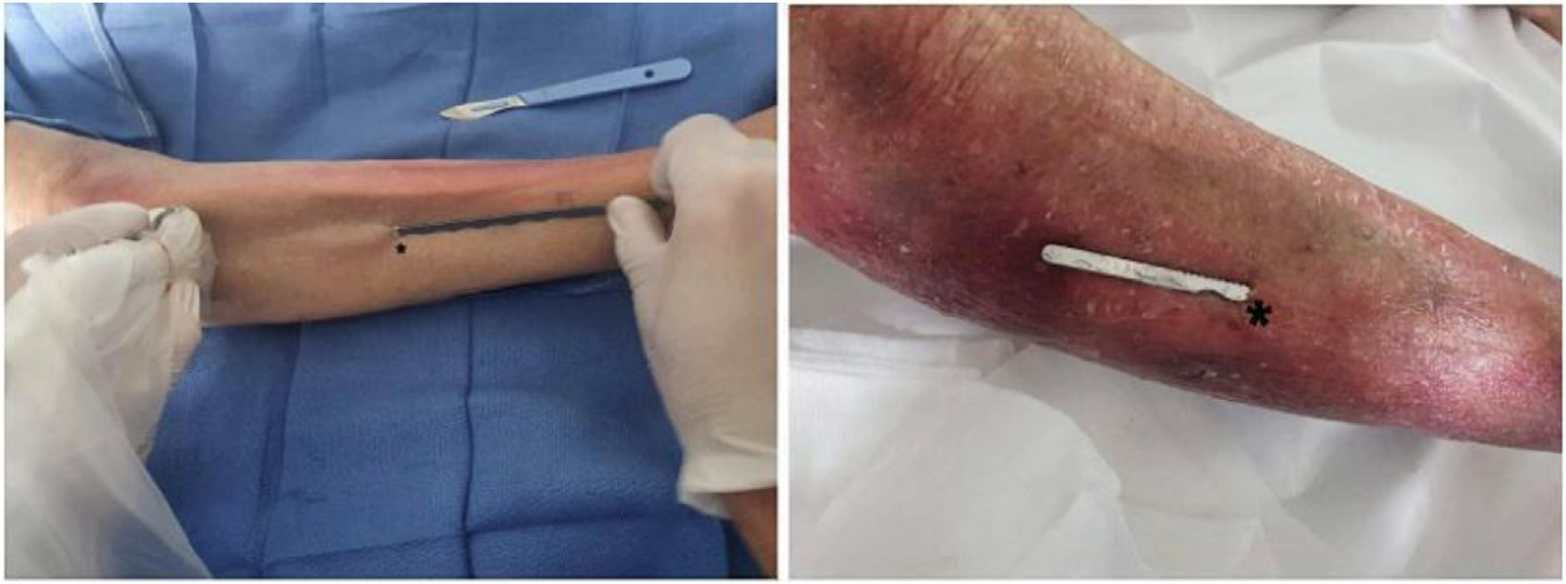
Intraoperative images of right leg showing use of ultrasound guidance while performing proximal division of fascia over anterior compartment. The asterisks indicate the minimally invasive incision.
